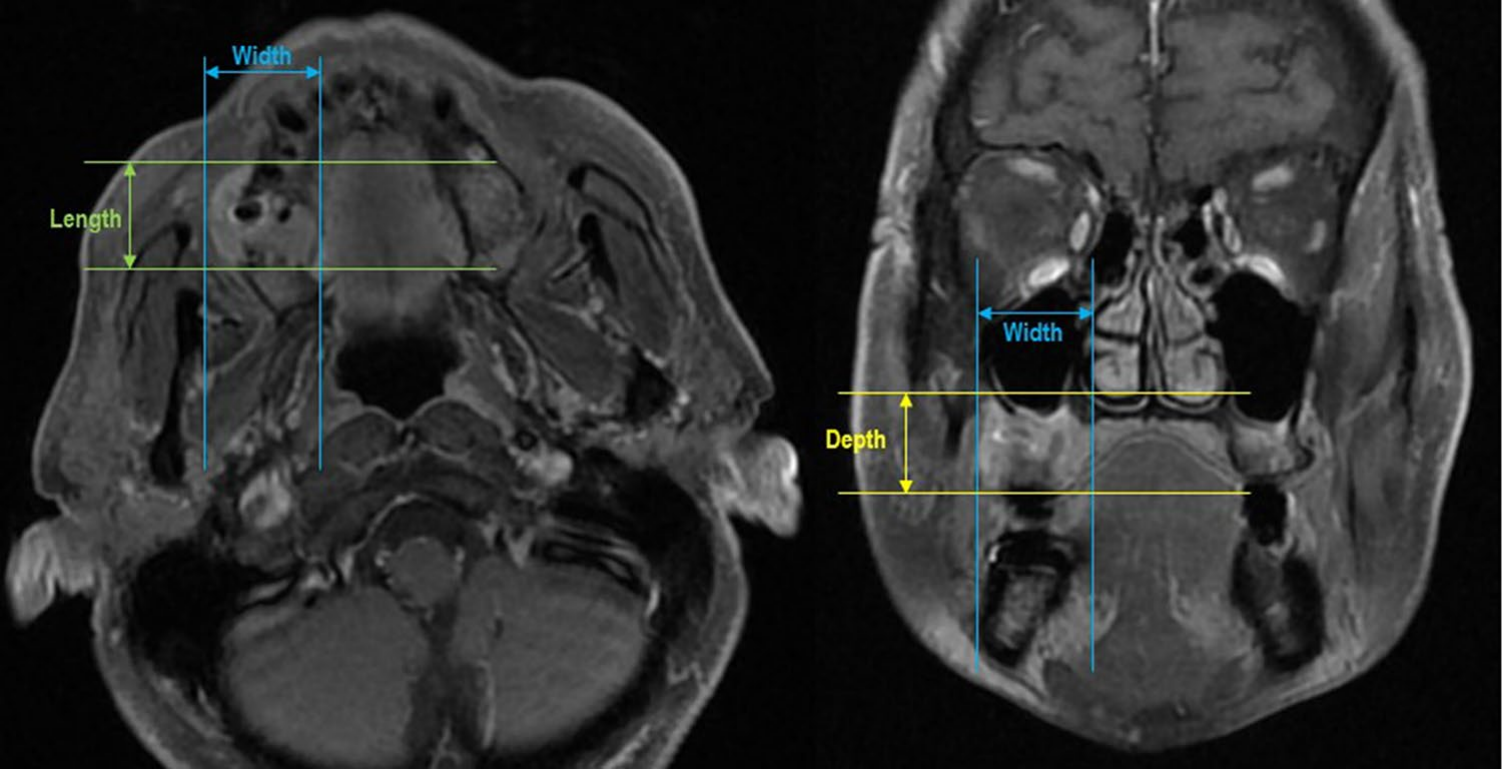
Orthogonal measurement of tumor size—non-metastatic patient. Tumor length and width were measured on axial MRI (left). Tumor depth and width were measured on coronal MRI (right). Both images were taken in a same patient where LN metastasis was NOT found
